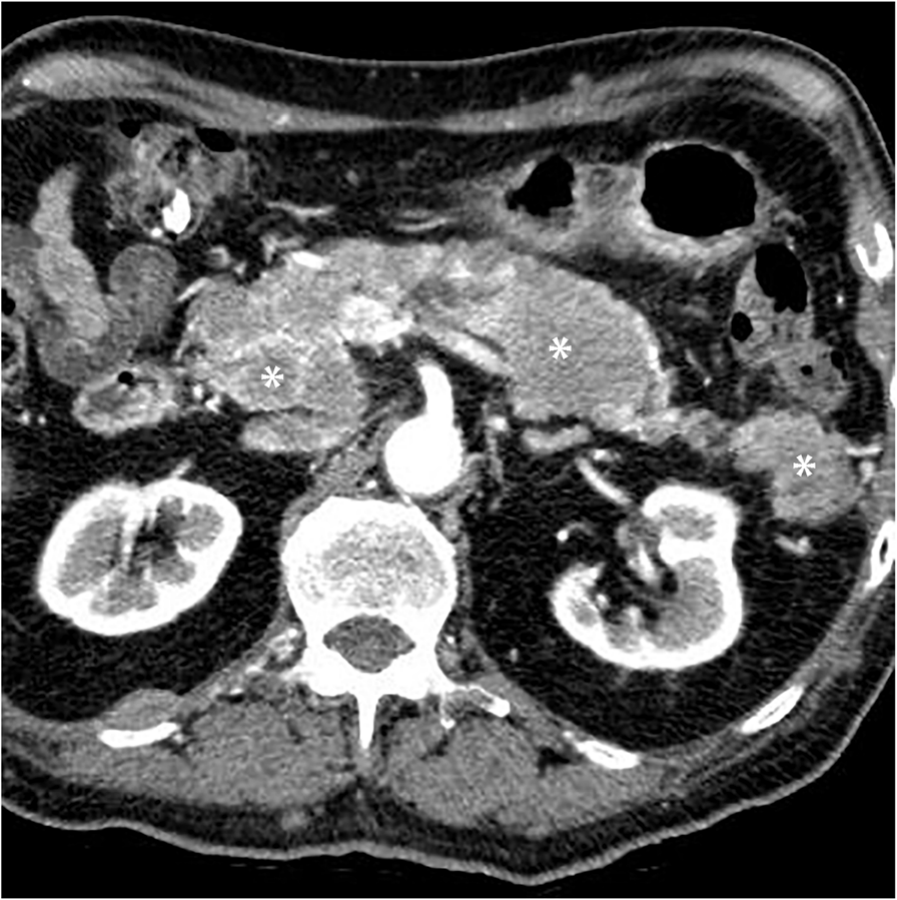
A 72-year-old man with papillary thyroid cancer metastasis involving the pancreas. Axial pancreatic phase CT image shows multiple enhancing masses (asterisks) involving the entire pancreas
